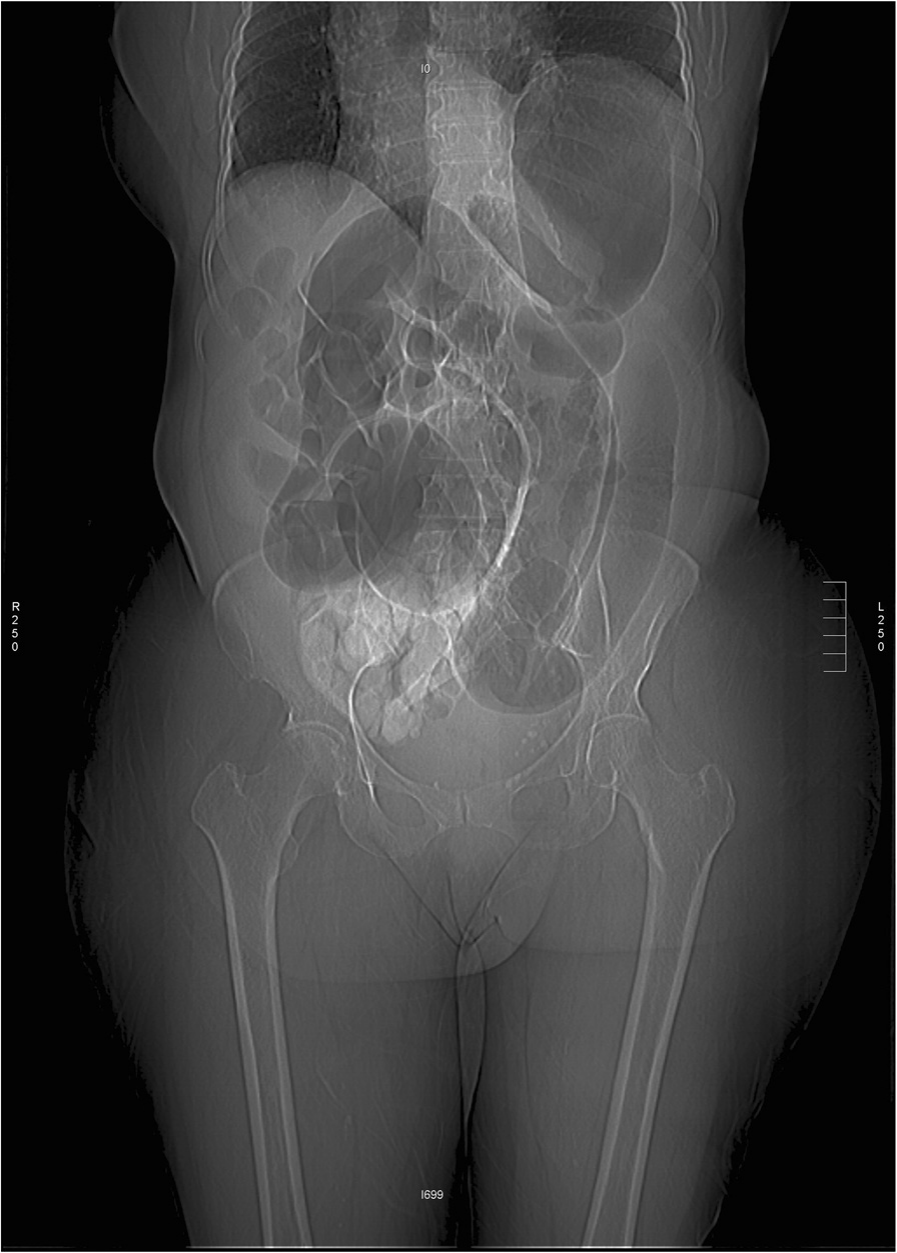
Radiograph demonstrating sigmoid volvulus.
